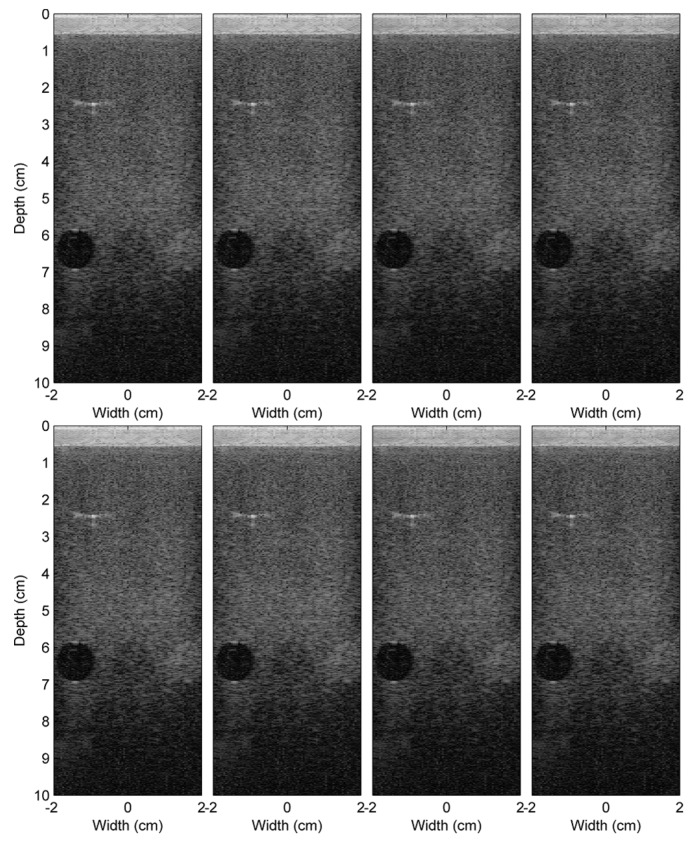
Comparison of the ultrasound images (from Experiment 1) reconstructed using eight different schemes, C-N-D, C-IQ-D, P-N-D, P-IQ-D, C-N-single (S) , C-IQ-S, P-N-S and P-IQ-S, from the left to the right and from the top to bottom, respectively.
